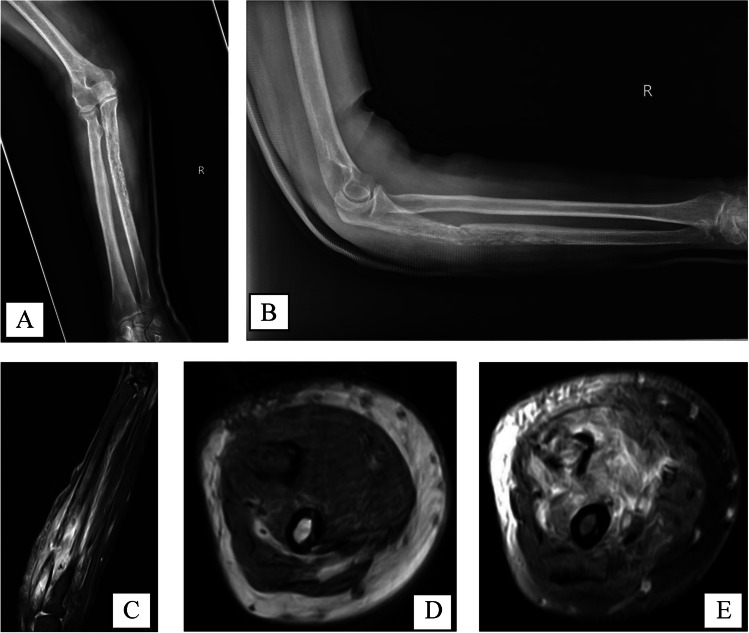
4/2021 — AP (A) and lateral (B) right forearm radiographs demonstrating an infiltrative lesion along the proximal to mid-ulnar diaphysis with associated minimally displaced angulated pathologic fracture. Coronal FS PD FSE (C), axial T1 (D), and FS PD FSE (E) MRI sequences showing complete destruction of the ulnar cortex with diffuse circumferential fluid hyperintensity in the surrounding soft tissues consistent with surrounding edema
